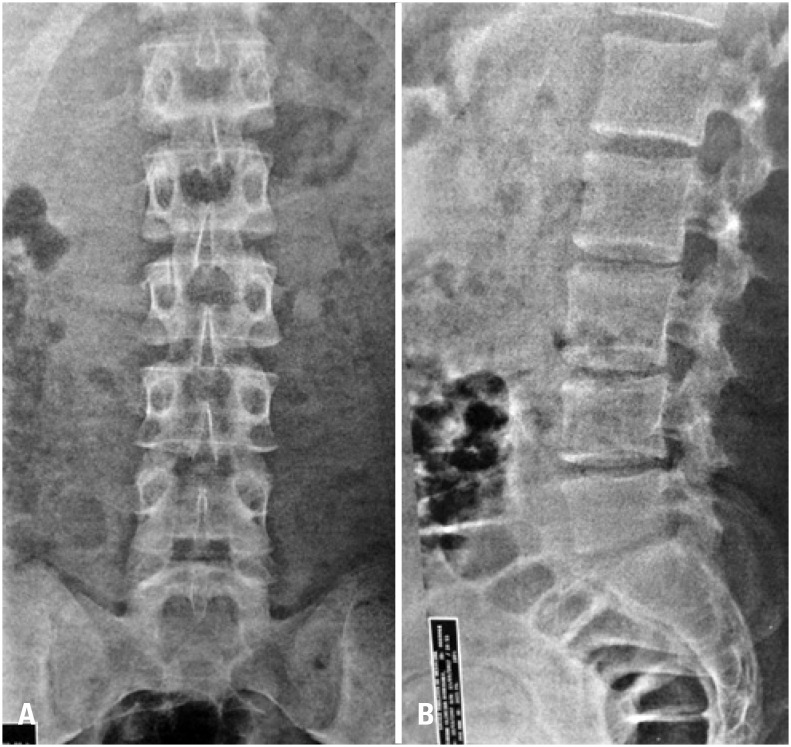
(A) Posteroanterior radiographs of the lumbar sacral spine. (B) Lateral radiograph of lumbar sacral spine
